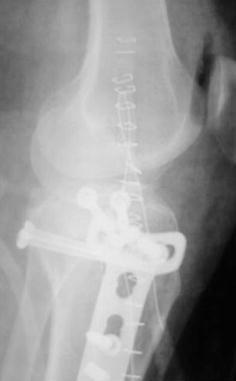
Schatzker type V fracture, post-op X-rays (LL)
